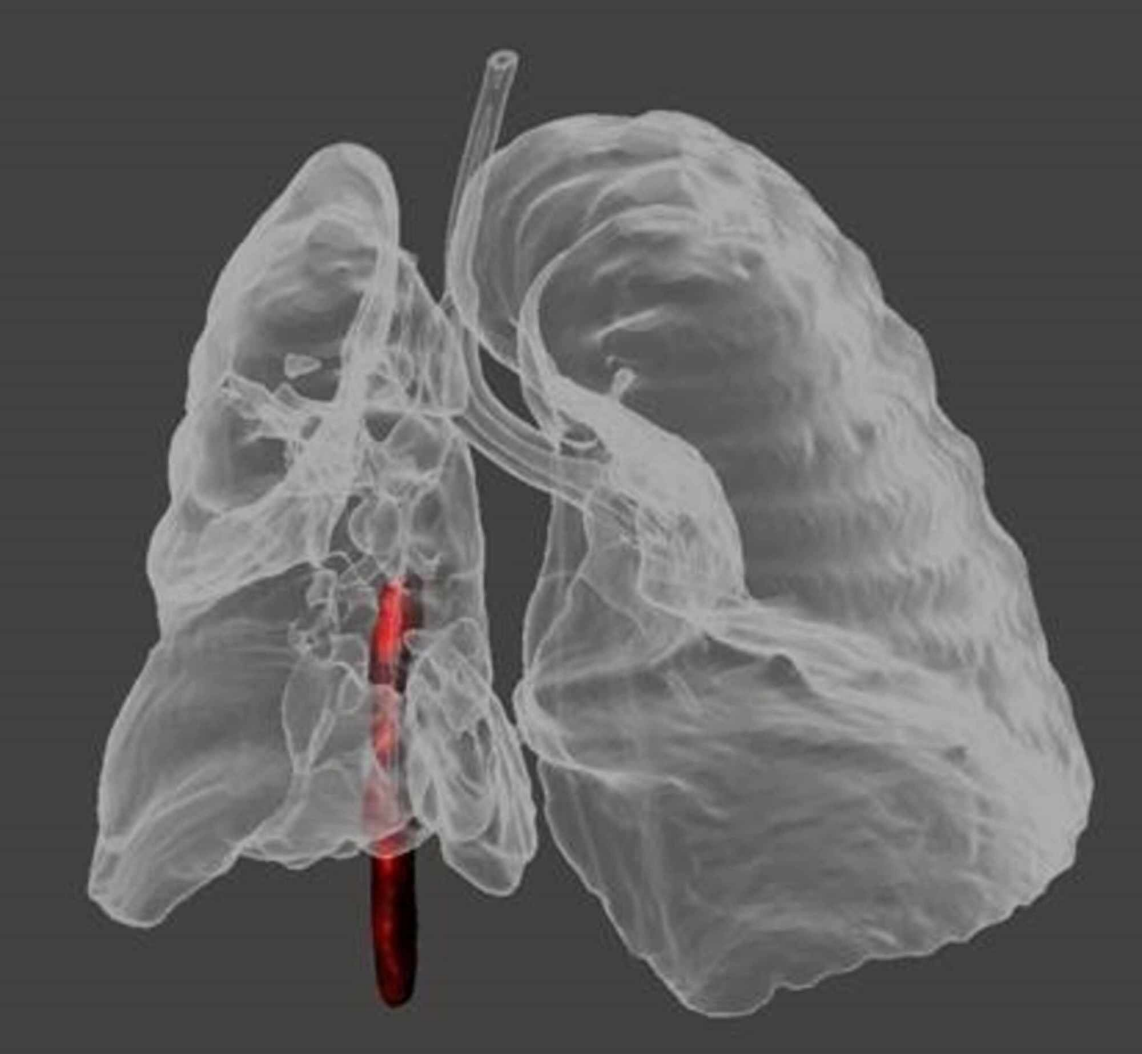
3D view of the chest CT - frontal projection Frontal projection of the 3D reconstruction of neonate's chest CT prior to removal of the foreign body (shown in red) CT: computed tomography
